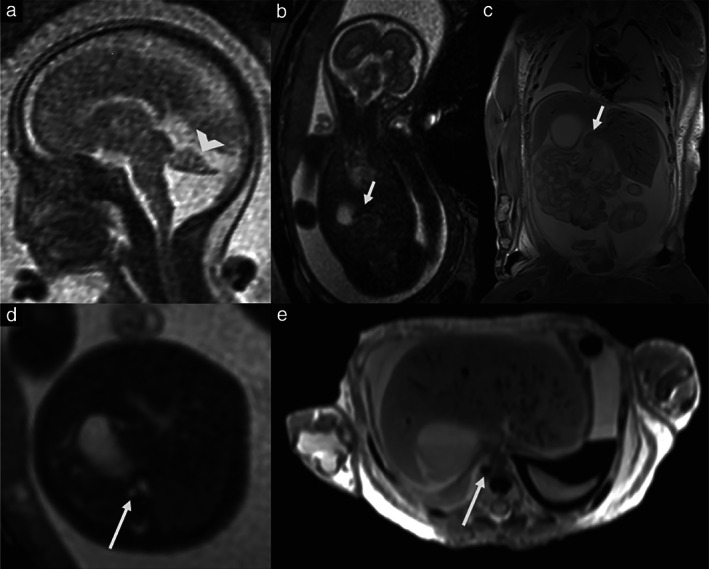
Magnetic resonance images in a fetus with left atrial isomerism, obtained at 23 + 0 weeks' gestation (a,b,d) and postmortem at 23 + 4 weeks (c,e), showing Dandy–Walker malformation with vermian hypoplasia and intestinal malrotation (arrowhead) (a), abnormal liver configuration with an additional liver lobule (short arrow) (b,c) and an azygos vein with interruption of the inferior vena cava (long arrow) (d,e).
